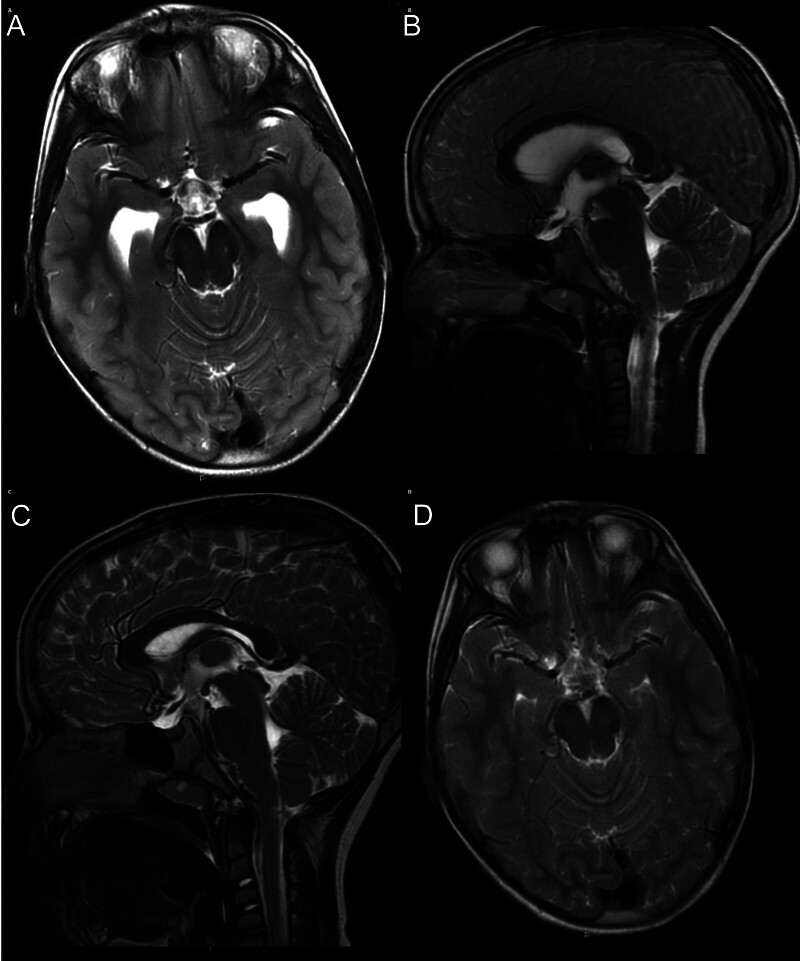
pre-and postoperative MRI demonstrating resolution of obstructive hydrocephalus caused by an intraventricular arachnoid cyst. (
